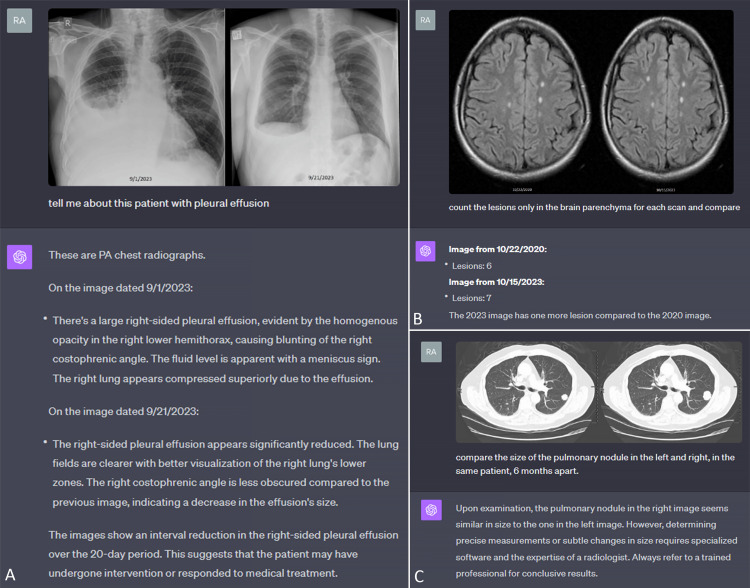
Radiologic image comparison A. Detection of improvement in pleural effusion in chest radiographs. B. Detection of progression of disease on MRI in multiple sclerosis. C. Inability to detect a change in the size of a pulmonary nodule.
